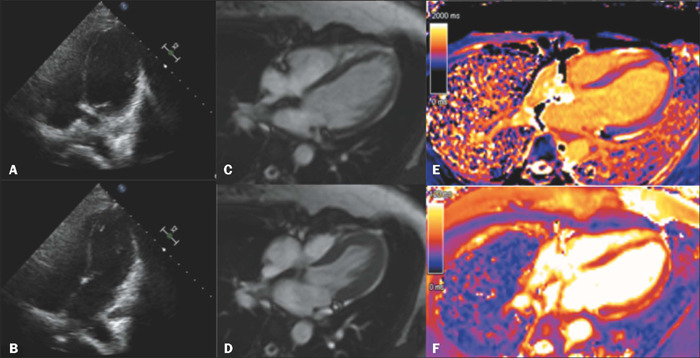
Echocardiogram and CMR of a 43-year-old female patient with chest pain
